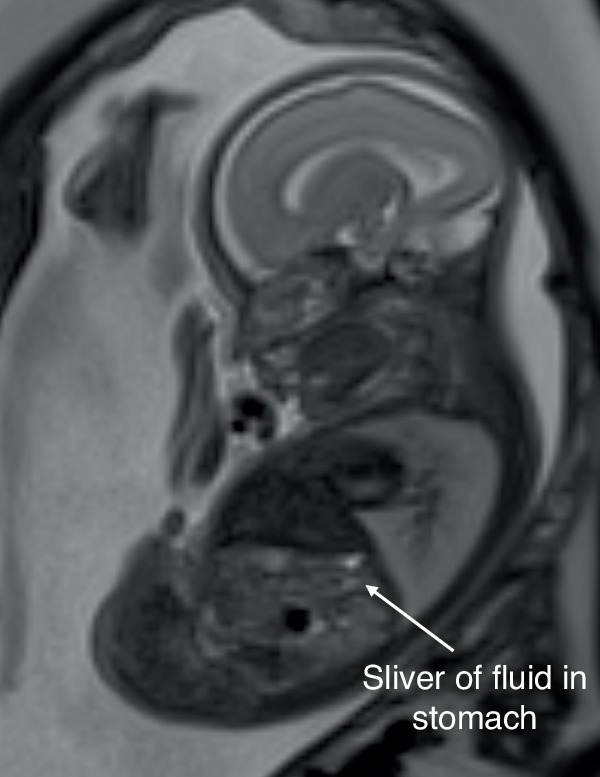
Sliver of stomach fluid. Sagittal T2 SSFSE fetal MRI image of a 25-week fetus with a sliver of fluid visible in the stomach.
